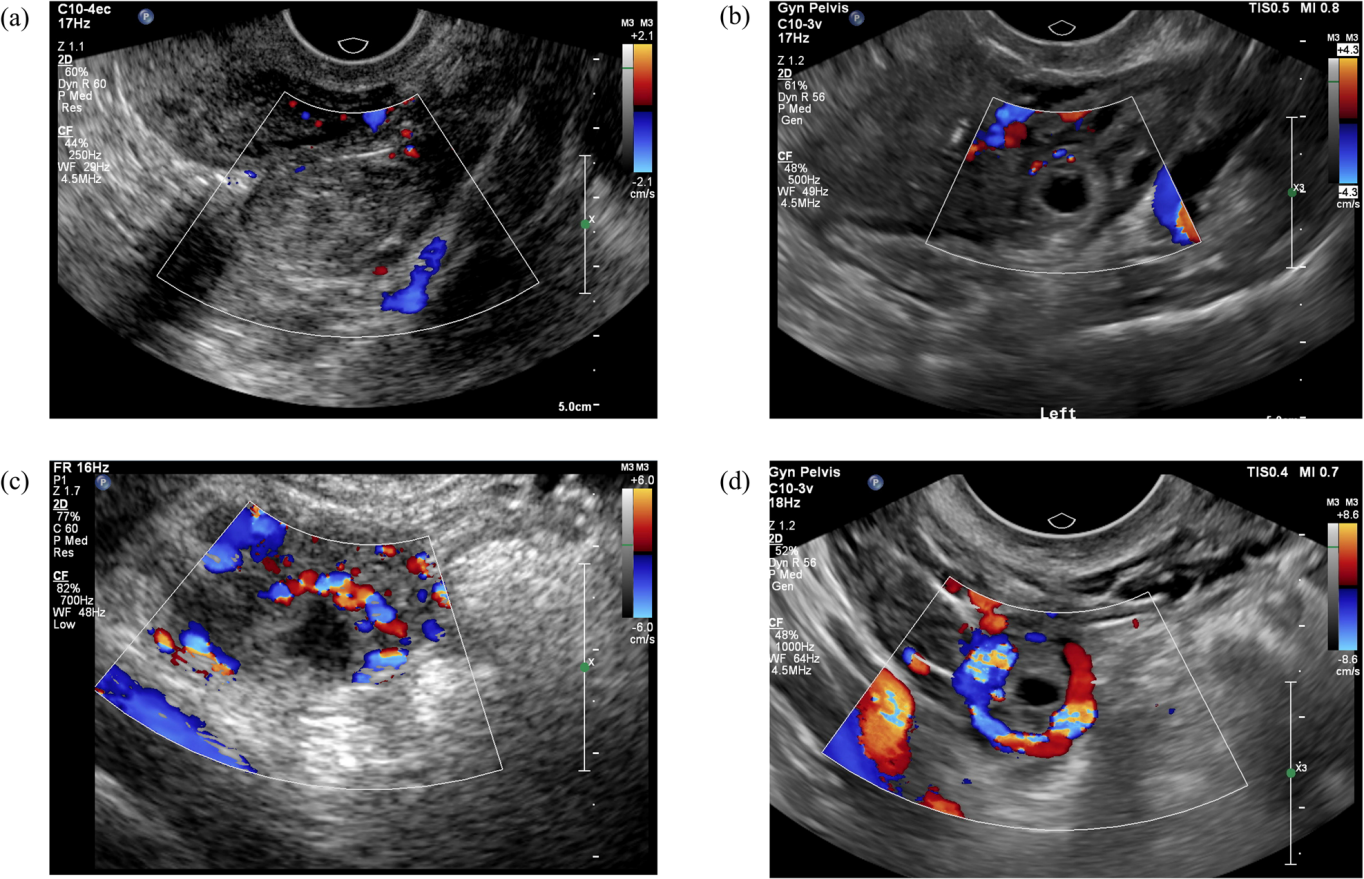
Color Doppler flow image grading in tubal ectopic pregnancies. (a) grade 0: no vascularity; (b) grade 1: vascularity occupying less than one third of the trophoblastic ring or suspected pregnancy mass; (c) grade 2: vascularity occupying more than one third but less than two thirds of the trophoblastic ring or suspected pregnancy mass; (d) grade 3: vascularity occupying more than two thirds of the trophoblastic ring or suspected pregnancy mass. The example images provided here were all obtained via transvaginal ultrasound
